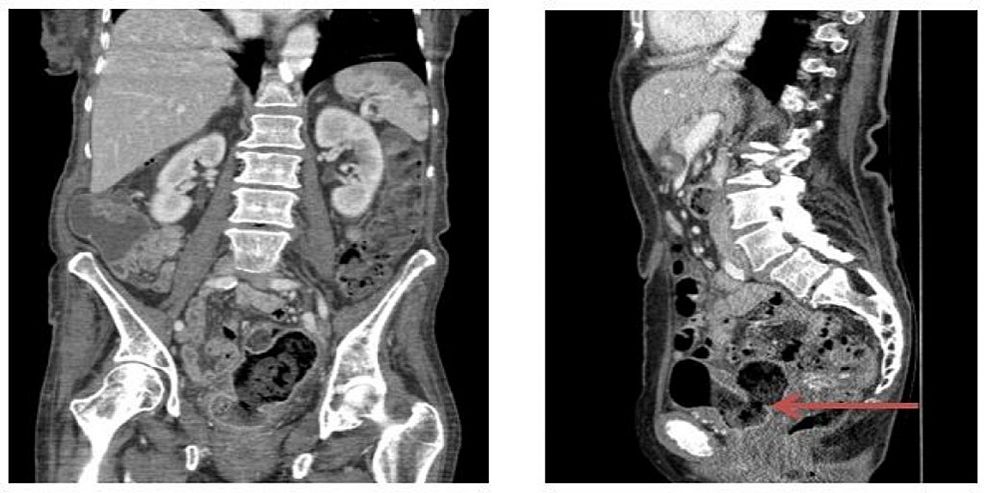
Contrast-enhanced CT-abdomen and pelvis (portal venous phase): coronal and sagittal views. The wide-necked connection between the proximal sigmoid and the bladder with extensive faecal residue filling the bladder, consistent with a colovesical fistula.
